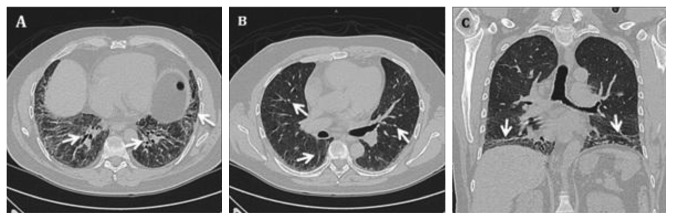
High-resolution computed tomography. (a) Bilateral pulmonary posterior basal sections showing a reticular pattern characterized by septal fibrosis and traction bronchiectasis. (b) At the carinal bifurcation region, a micronodular image is visible along with multiple subpleural ground-glass opacification zones. (c) Coronal plane evidence of fibrosis located in both lung bases.
